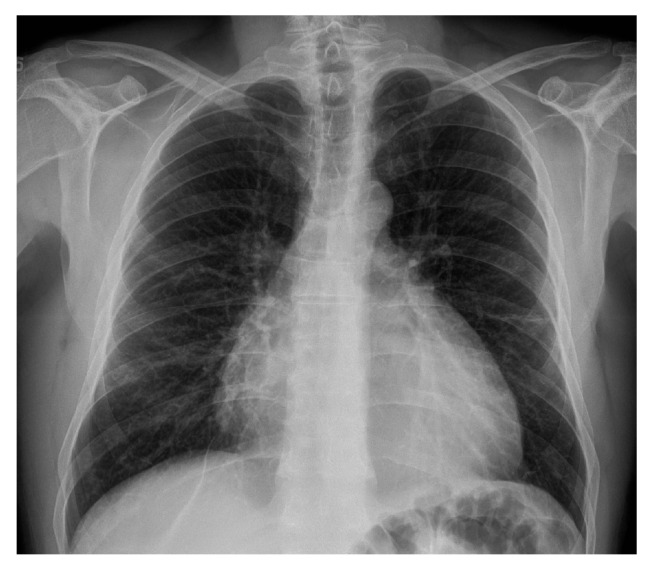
Chest X-ray. PA projection. Global cardiomegaly with rounded cardiac shape. No opacities were observed.
